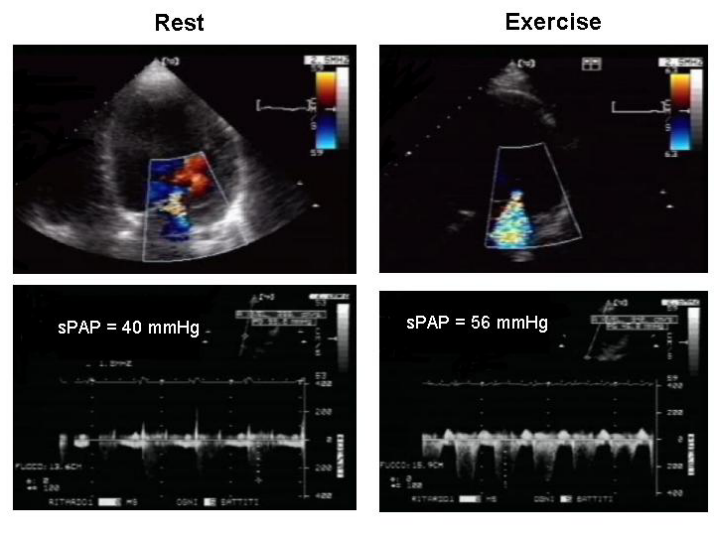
Apical four-chamber view at rest and during exercise in patients with ischemic mitral regurgitation showing a large exercise-induced increase in mitral regurgitation. SPAP = Systolic pulmonary pressure.
